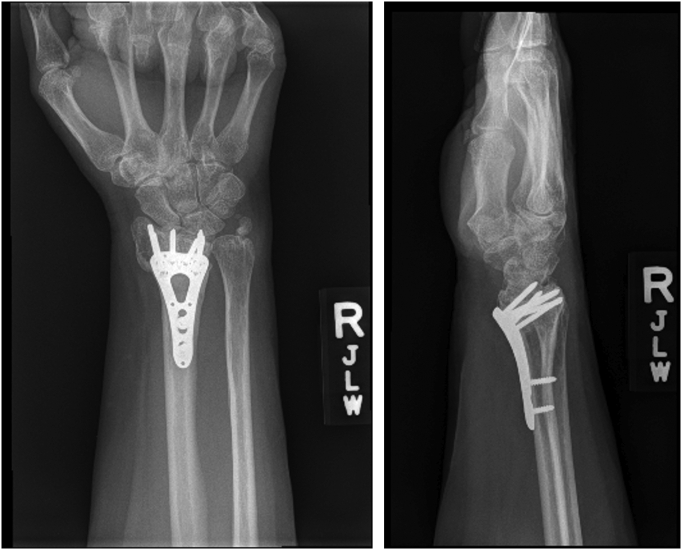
Six-month postoperative radiographs demonstrating intact distal radius hardware with no evidence of loosening.
